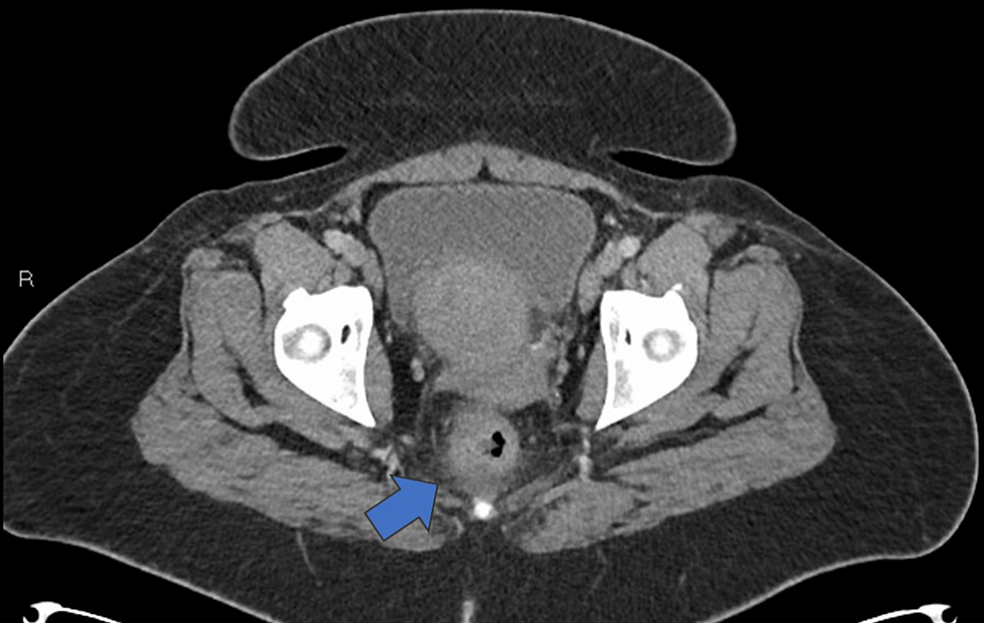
CT of the abdomen and pelvis on admission showing proctocolitis in the axial view (blue arrow)
